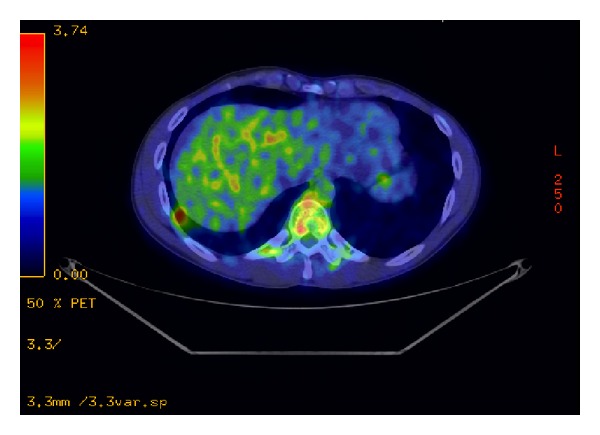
PET CT scan shows hypermetabolism of lung base nodule.
